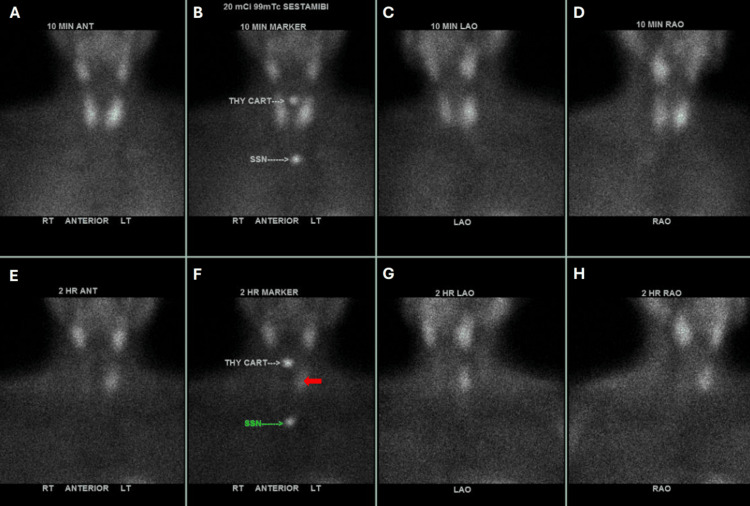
Technetium-99m (99mTc) sestamibi parathyroid scintigraphy (A-D) Early 10-minute planar images. (E-H) Delayed two-hour planar images demonstrating near-complete thyroid washout with persistent focal radionuclide retention (red arrow) posterior to the left thyroid lobe, most prominent in panel F, consistent with a left parathyroid adenoma. White arrows indicate anatomic landmarks. THY CART: thyroid cartilage, SSN: suprasternal notch, RT: right, LT: left, ANT: anterior, LAO: left anterior oblique, RAO: right anterior oblique
